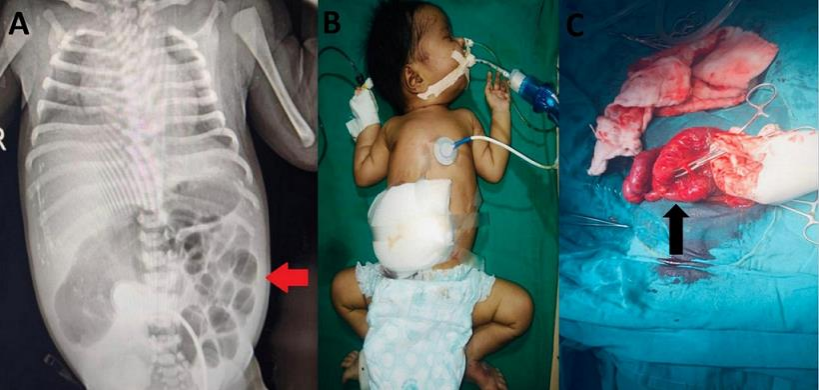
A) X-ray of the erect abdomen showing distended bowel loops and bilaterally elevated diaphragm; B) neonate with abdominal distention; C) the dilated small bowel proximal to volvulus (arrow) was resected
